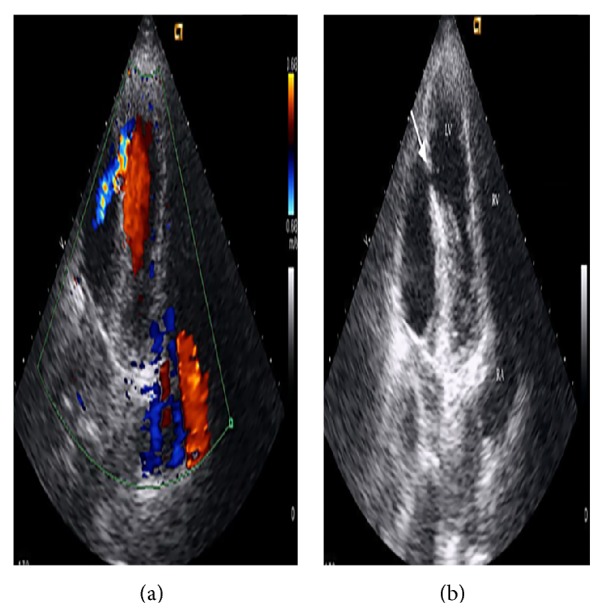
Modified apical four-chambered view in transthoracic echocardiography showing the defect (b) (marked by white arrow) and color jet (a) through it. LV: left ventricle, RV: right ventricle, RA: right atrium, and IMD: intramyocardial dissection area.
